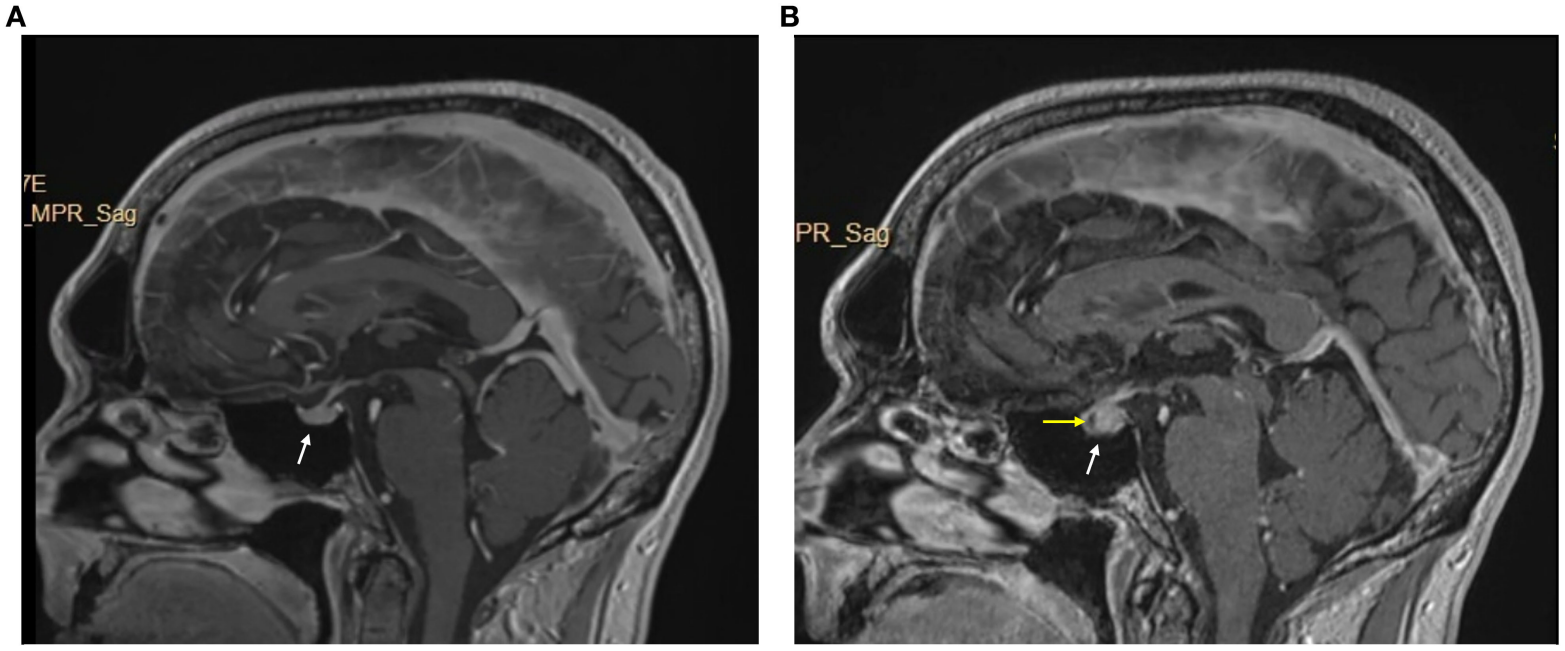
Brain MRI w/wo contrast pre- and post-immunotheraphy. (A) pre-immunotheraphy initiation; (B) post-immunotheraphy initiation.
